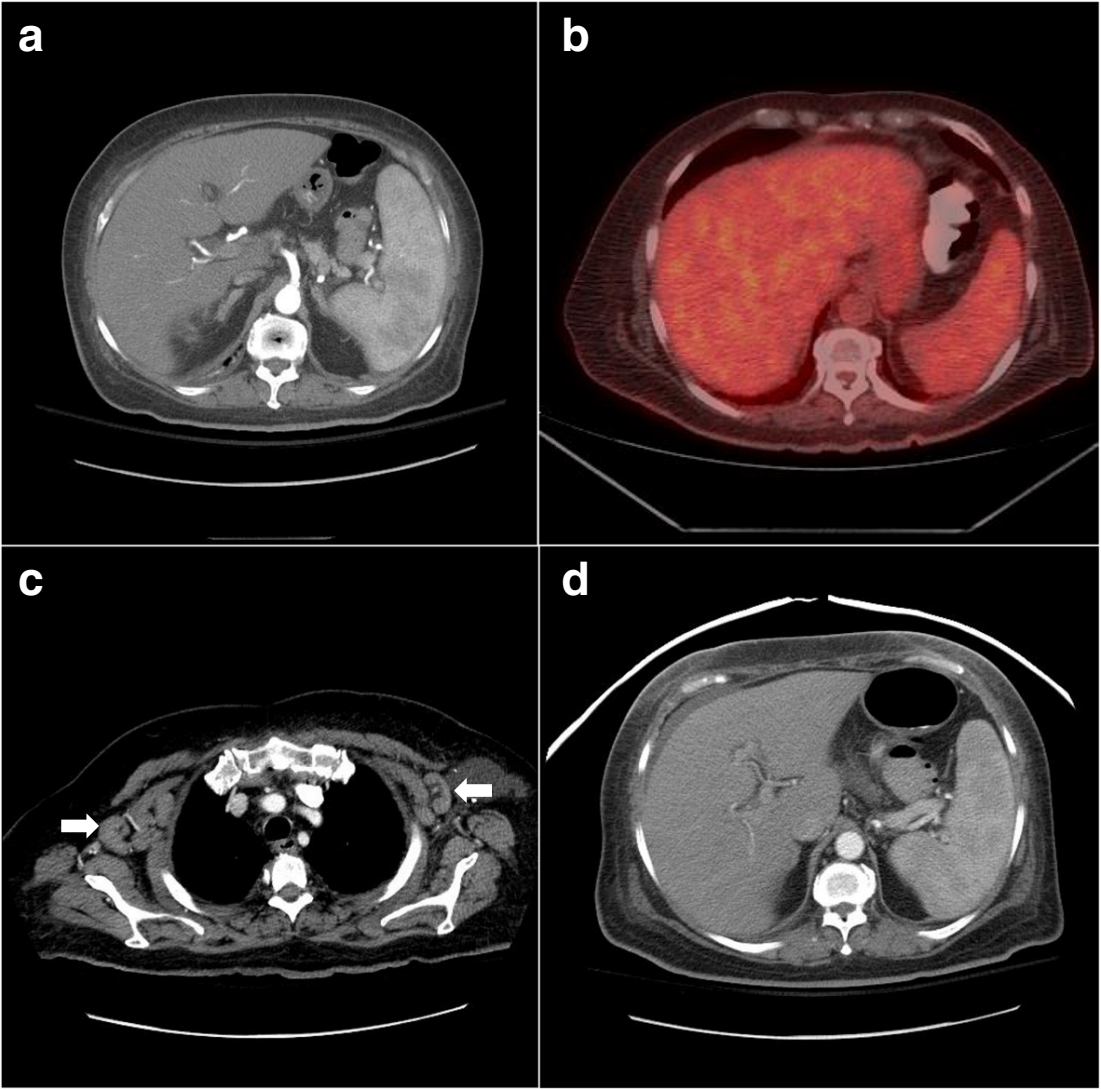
Imaging findings at disease presentation and progression. a CT abdomen and pelvis with contrast showing an enlarged spleen with micronodular pattern. b PET-CT imaging showing interval resolution of splenomegaly and documenting no evidence of hypermetabolic adenopathy. c Repeat CT chest with contrast evidencing development of a right axillary node measuring 2.9 × 2.4 cm and a left axillary node measuring 3 × 1.5 cm (white arrows). d Repeat CT abdomen and pelvis evidencing again an enlarged spleen with numerous small subtle hypodense nodules
